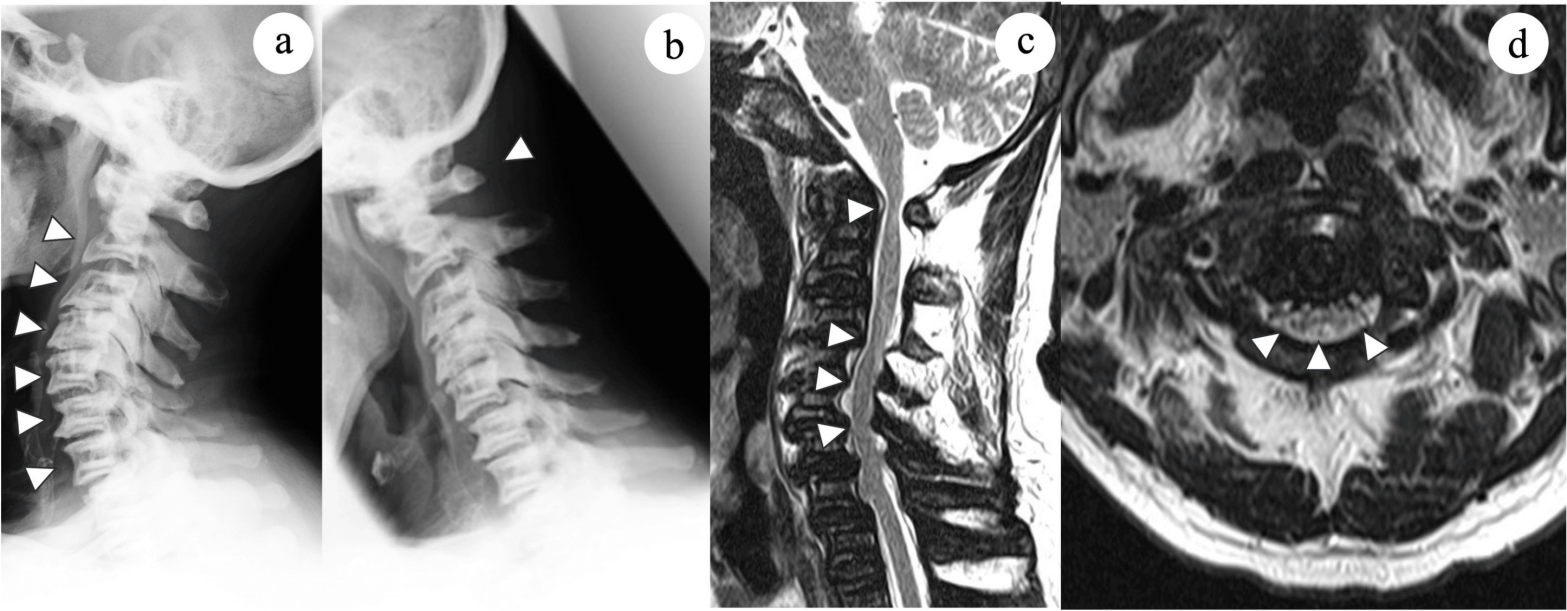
Radiological findings before surgery. (a, b) Plain radiographs showing sclerosis of the vertebral endplates and atlantoaxial subluxation (arrowheads). (c) Sagittal and (d) axial T2-weighted magnetic resonance images reveal spinal cord compression at C1 and C4–6 (arrowheads) with the bone marrow space showing a lack of signal alternating with a signal pattern similar to that of the intervertebral discs, giving a “stepladder” appearance.
